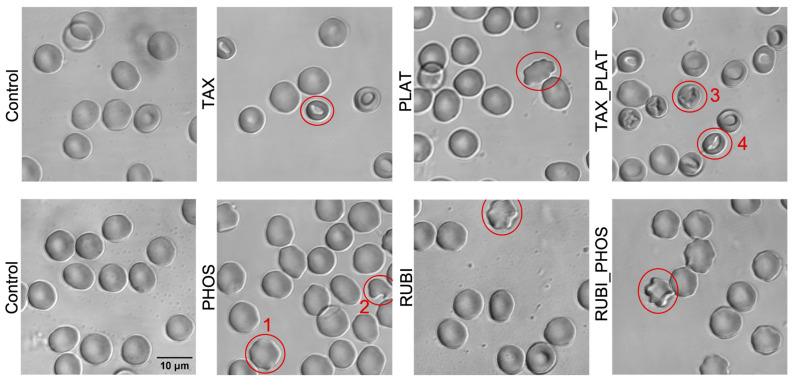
Disturbance of erythrocyte morphology under the action of CT drugs. Representative pseudocoloured confocal images (Leica TCS SP5 MP) of non-fixed RBCs show the appearance of anisocytosis and the disruption of RBCs’ native morphology (red circled RBCs as an example): control cells had the normal shape of discocytes, TAX—discocytes and stomatocytes I–II, PLAT—discocytes and echinocytes I, PHOS—discocytes, echinocytes I (1) and schistocytes (2), RUBI—discocytes and echinocytes I; the combinations of drugs intensified the change in morphology of RBCs: TAX_PLAT demonstrated discocytes and stomatocytes at different stages (3, 4) while RUBI_PHOS—discocytes and echinocytes II.
